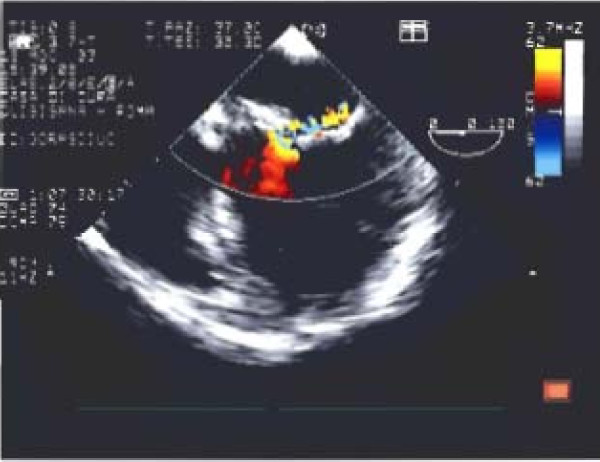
Transesophageal echocardiographic view of mitral valve: moderate-severe mitral regurgitation with jet originating centrally and directed towards lateral wall of the left atrium is seen.
